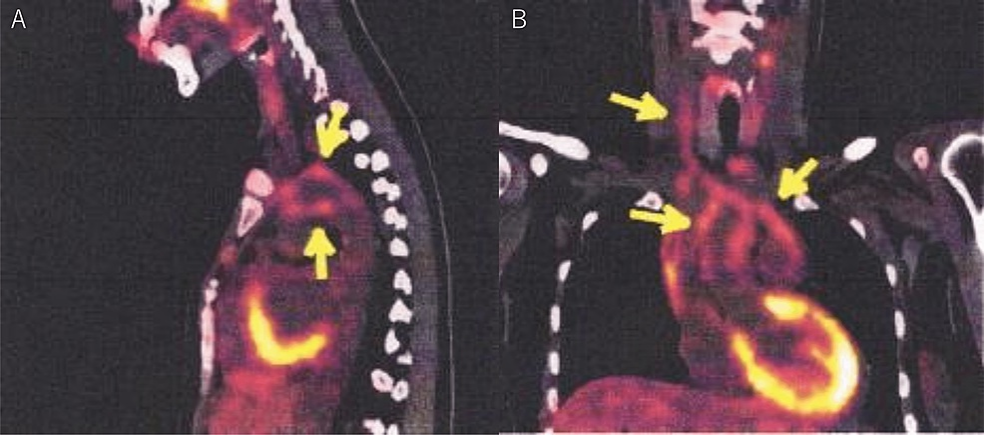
18F-fluorodeoxyglucose positron emission tomography. There was an accumulation of 18F-fluorodeoxyglucose in the vessel wall from the ascending aorta to the aortic arch (SUVmax 2.93-3.06) (yellow arrows on the aorta in A and B) and from the brachiocephalic artery to the right common carotid artery (SUVmax 2.53-2.56) (yellow arrow in the cervical area in B). SUV: standardized uptake value.
